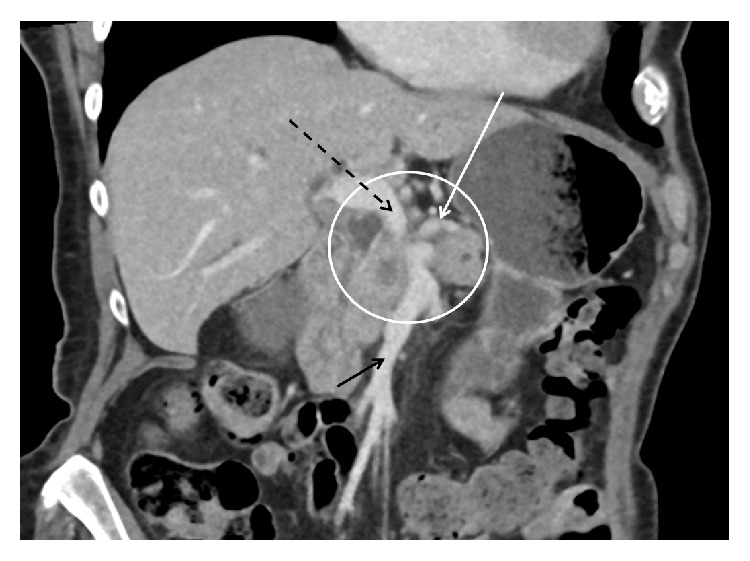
CT scan (coronary reconstruction) showing PDAC tumor infiltration of the portal vein confluence (white circle). Superior mesenteric vein (black arrow), portal vein (broken black arrow), and splenic vein (white arrow) without thrombosis, adequate diameter of the portal, and superior mesenteric vein to perform an end-to- end anastomosis.
